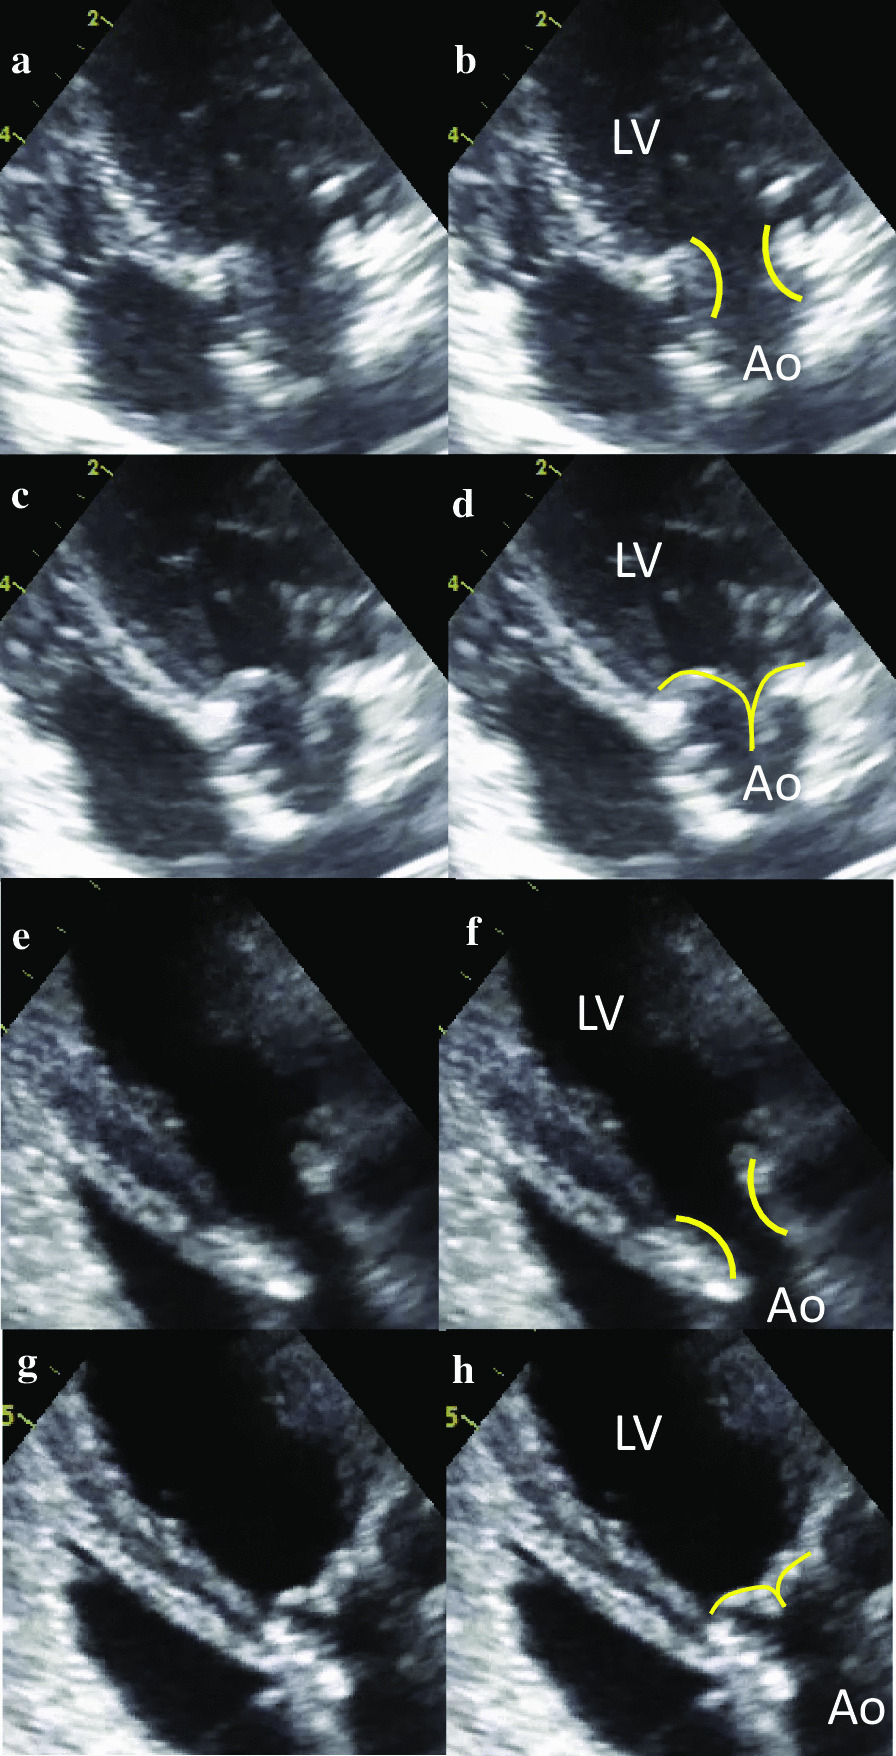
Movement of the leaflets in the aortic valve position by echocardiography 3 months after implantation. Both biosheet (systolic; a, b and diastolic; c, d) and autologous pericardium (systolic; e, f and diastolic; g, h) valve leaflets were smoothly opening and closing. Yellow lines show leaflets. The visible thrombus and cuspal tears were not detected. LV left ventricle, Ao aorta
